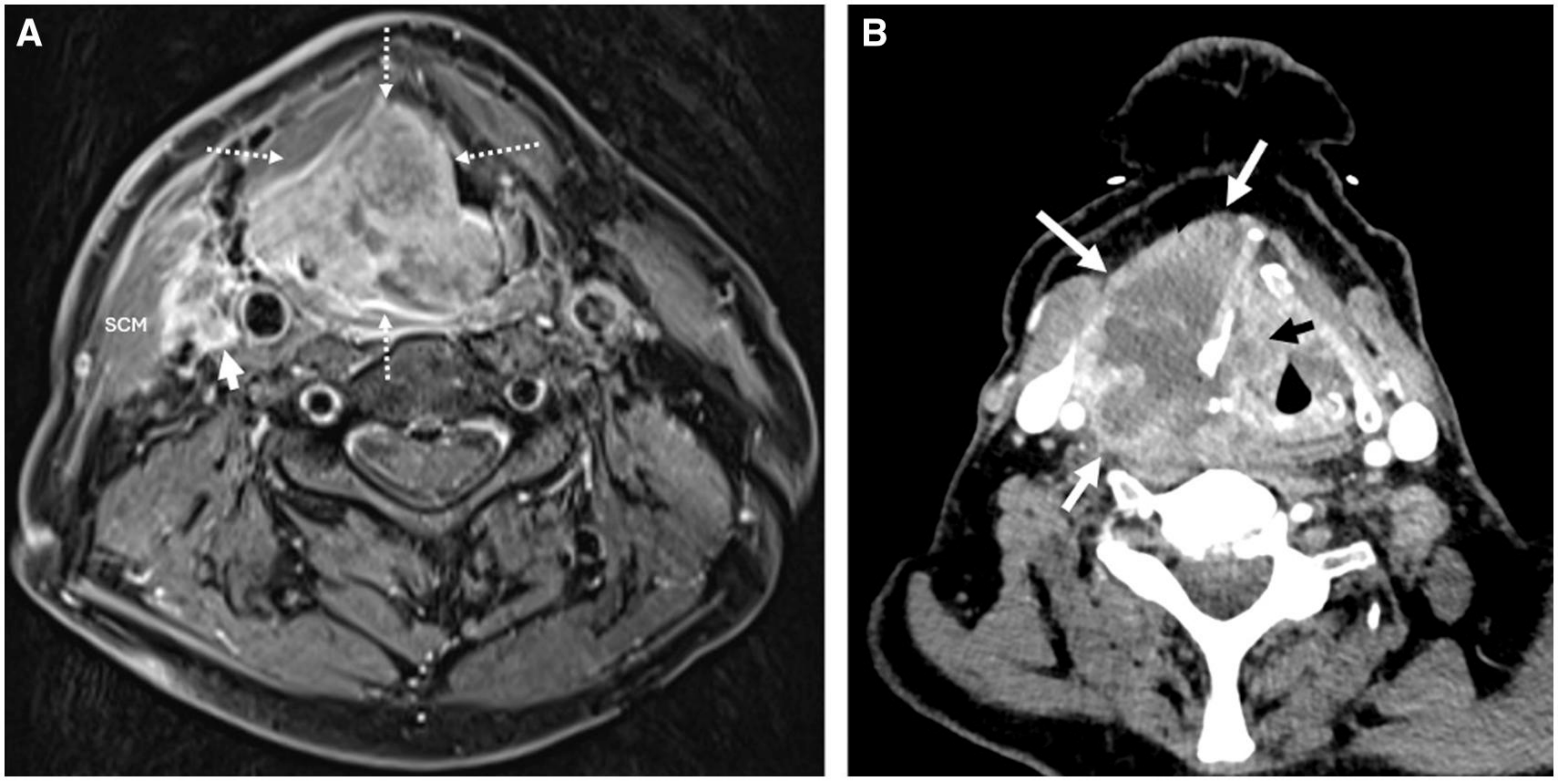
(A) Transglottic SCC: axial post-contrast fat-suppressed T1-weighted MRI shows a bulky right laryngeal tumour (dotted white arrow) with internal necrosis (intralesional areas of non-enhancement) and ipsilateral necrotic level 3 nodal metastasis (short solid white arrow).The metastatic nodes are matted and show radiological features of extracapsular spread into the sternocleidomastoid muscle (SCM). (B) Anaplastic thyroid carcinoma: CECT shows a necrotic (centrally hypoattenuating) mass (solid white arrow) centred of the right lobe of the thyroid gland invading into the larynx (black short arrow).
